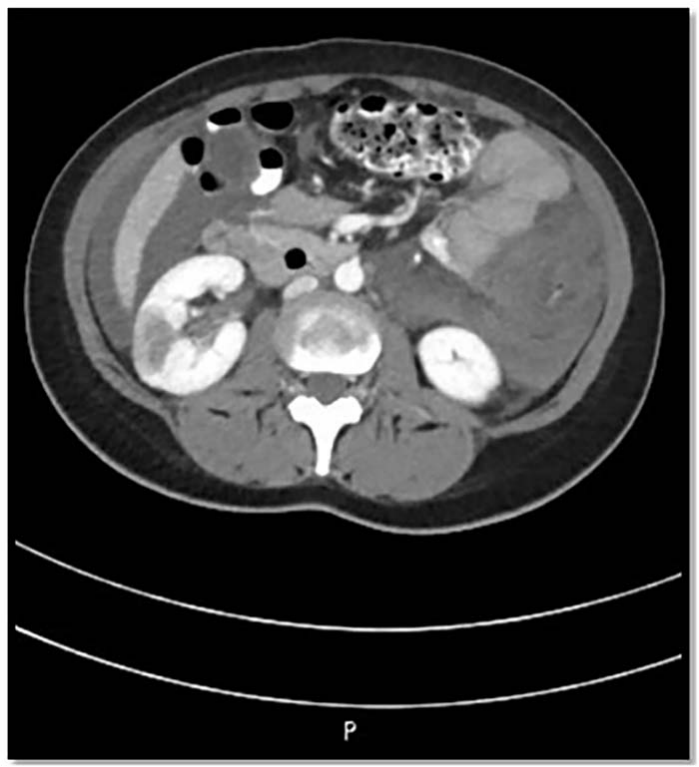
CT abdomen findings show significant thickening of the transverse colon with significant pericolic fat stranding; the right kidney shows wedge-shaped cortical hypodensities (could represent ischemic insult).
